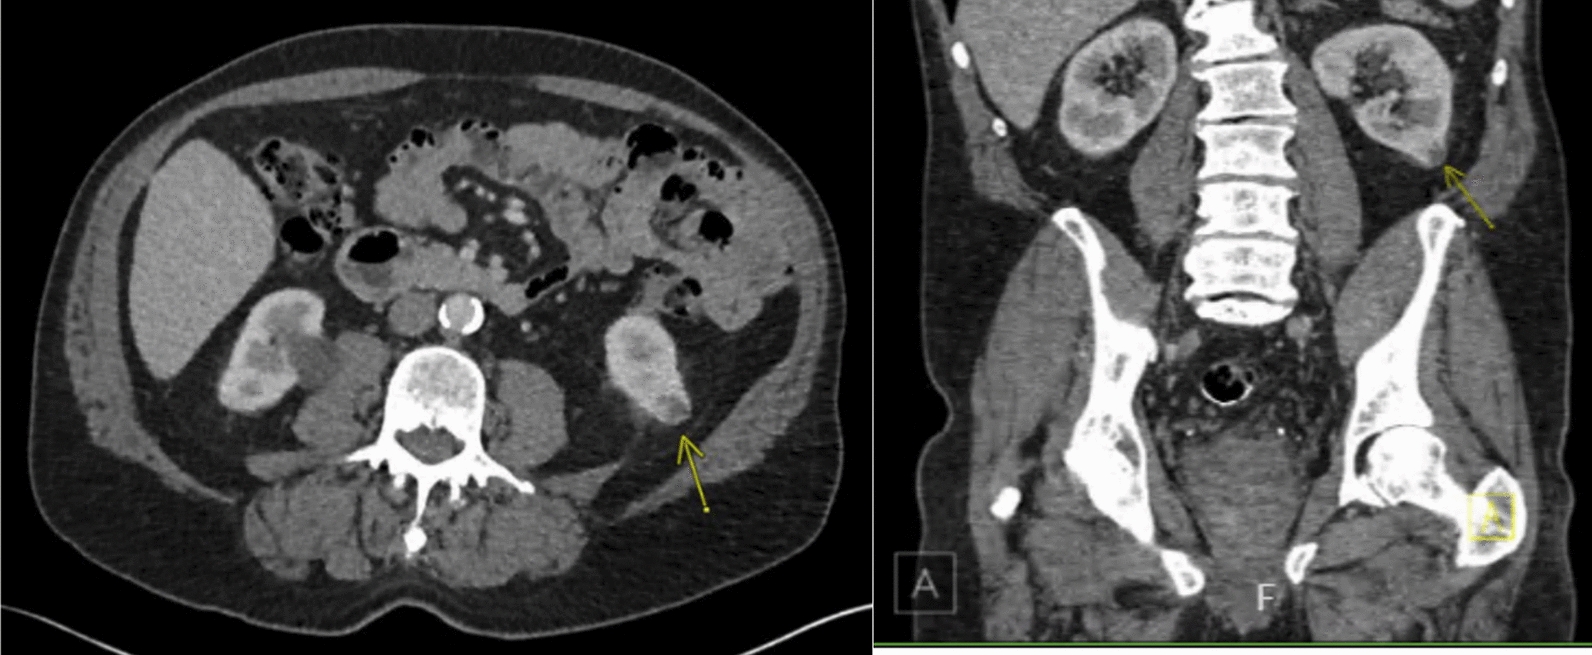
Case 1: Axial and coronal CT scan slices of biopsy-confirmed renal cell carcinoma
